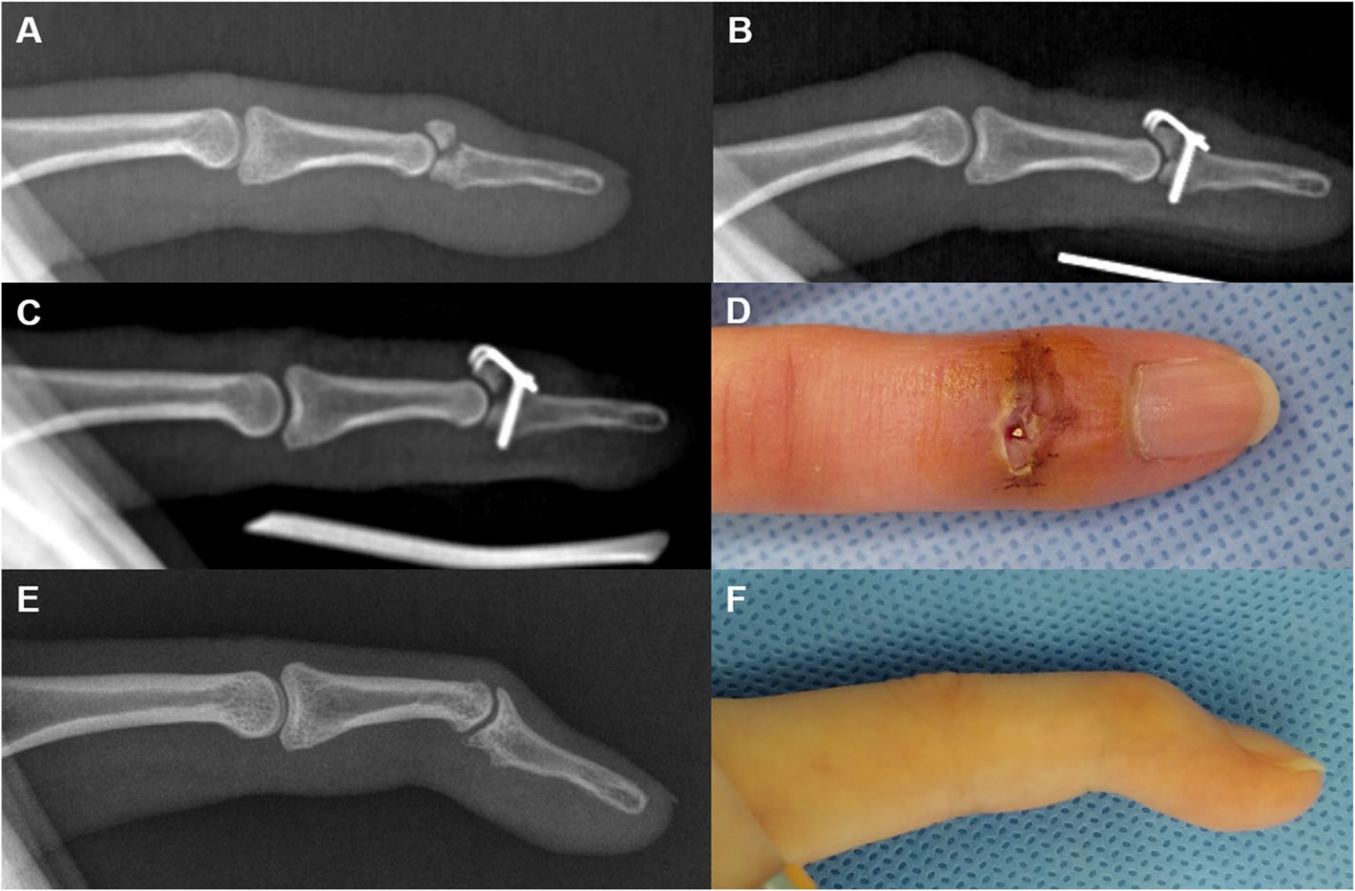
Mallet fracture (a). A mini-hook plate captured the dorsal avulsed fragment (b). Plain radiography 2-weeks post-operation demonstrated that the mallet fragment with the hook plate had slightly migrated proximally and dorsally (c). The plate was exposed to the dorsal skin, and the patient underwent revision surgery (extension block pinning) after plate removal (d). Plain radiography 57 months post-operation reveals that the osseous fragment was well united, but the articular surface was incongruent. The total range of DIP joint motion was 50%, and the pinching strength was 45% compared to the values of the same contralateral joint (e, f)
